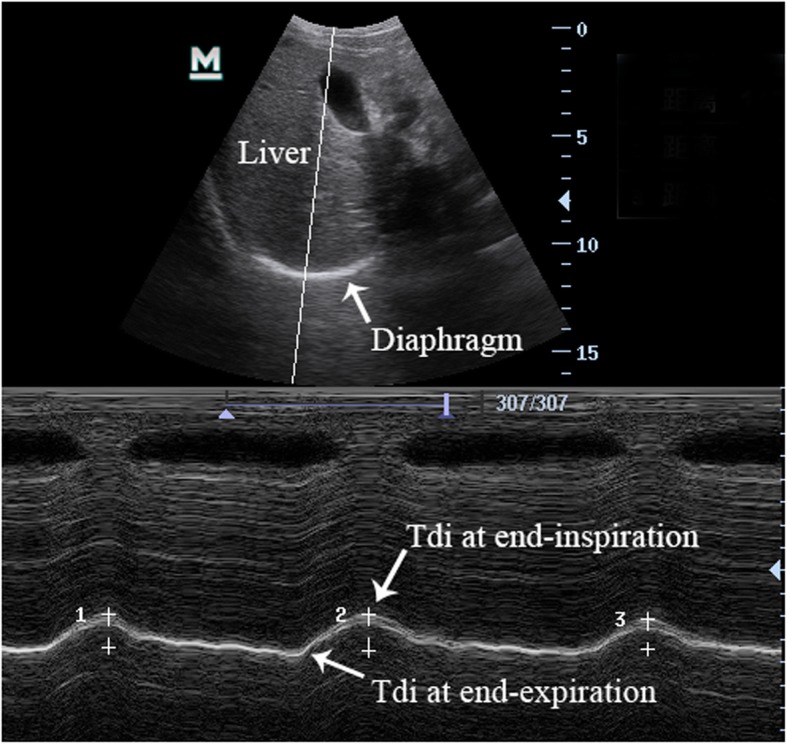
Ultrasound assessment of diaphragm diaphragmatic excursion in M-mode
